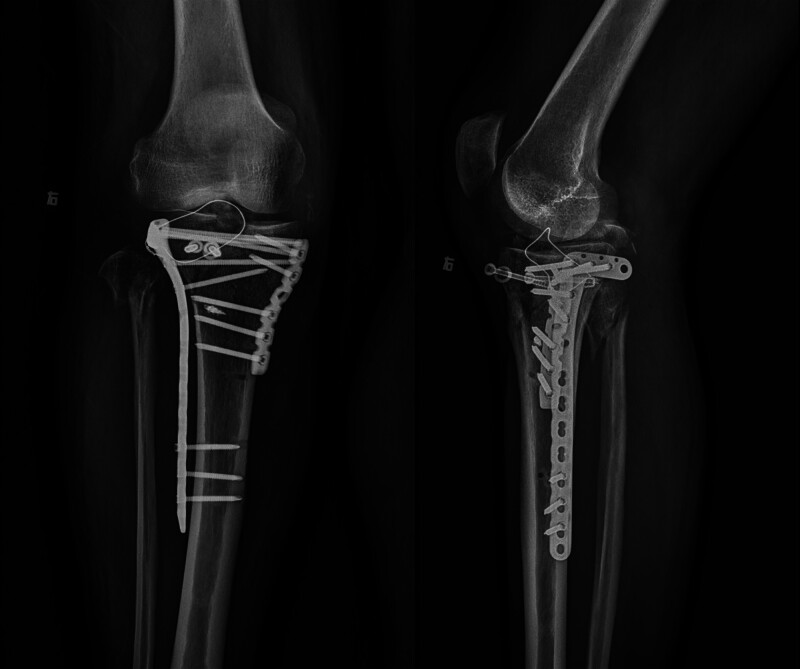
Postoperative radiographs showed fracture reduction was observed, and the implant position was similar to previous radiographs (Fig. 4; date of examination: September 25, 2021). (A) Plain x-ray of the knee with anteroposterior view. (B) Plain x-ray of the knee with lateral view.
